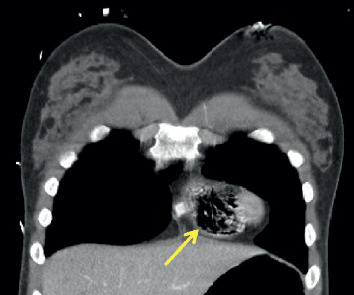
Coronal view of CT chest demonstrating significant amount of air detected in the right ventricle (arrow).
